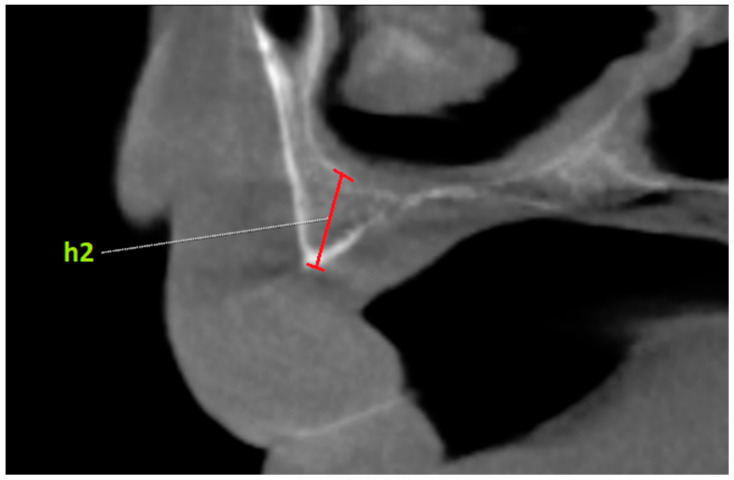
Measurement of the area where the virtual implant was placed in the para-axial section obtained from CBCT. h2: vertical height of the area between the lower border of the nasal cavity and the alveolar crest ridge.
